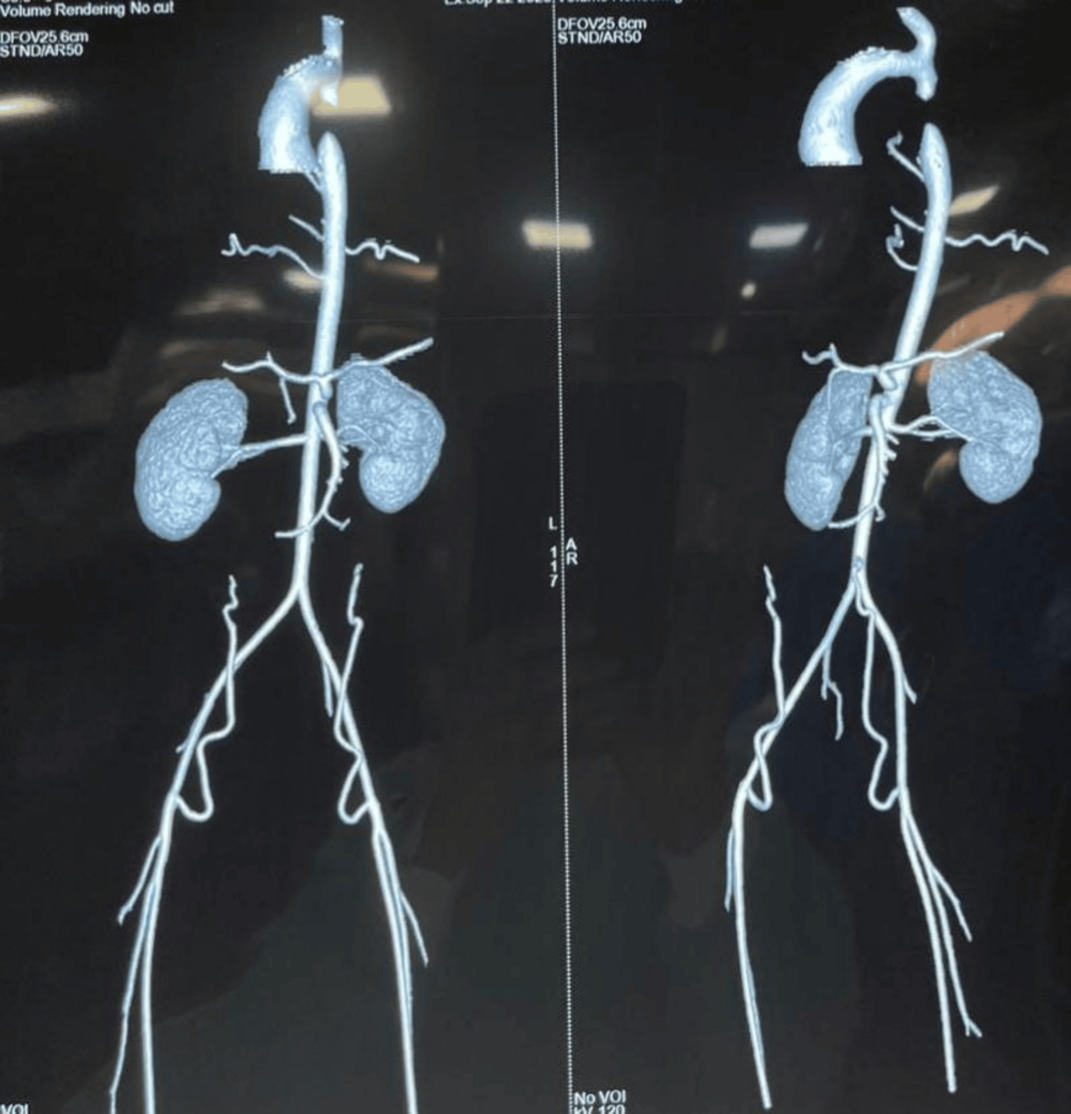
CT angiography of the aorta demonstrating severe coarctation of the aorta distal to the left subclavian artery
